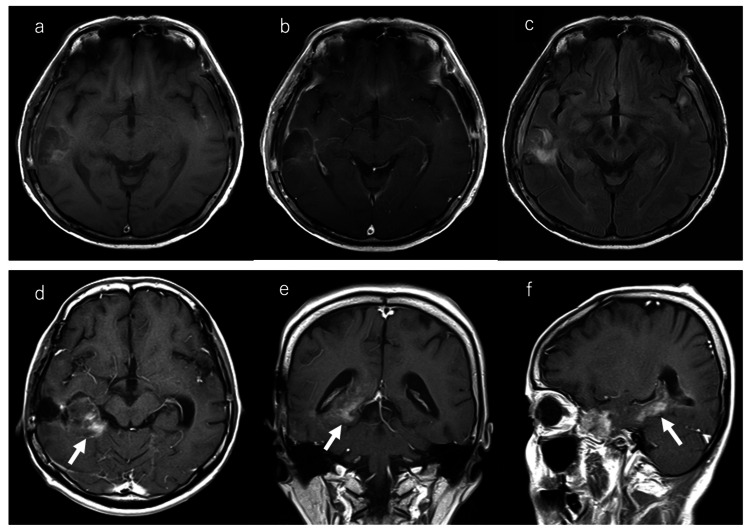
Postoperative MRI and recurrence MRI. (a) Postoperative T1-weighted MRI (axial). (b) Postoperative contrast-enhanced T1-weighted MRI. (c) Postoperative FLAIR image (axial). (d) Axial contrast-enhanced T1-weighted MRI at recurrence revealing a new ring-enhancing mass at the periphery of the right temporal resection cavity (white arrow). (e) Coronal contrast-enhanced T1-weighted MRI demonstrating the recurrent tumor (white arrow). (f) Sagittal contrast-enhanced T1-weighted MRI showing the recurrent tumor (white arrow). FLAIR: fluid-attenuated inversion recovery; MRI: magnetic resonance imaging.
